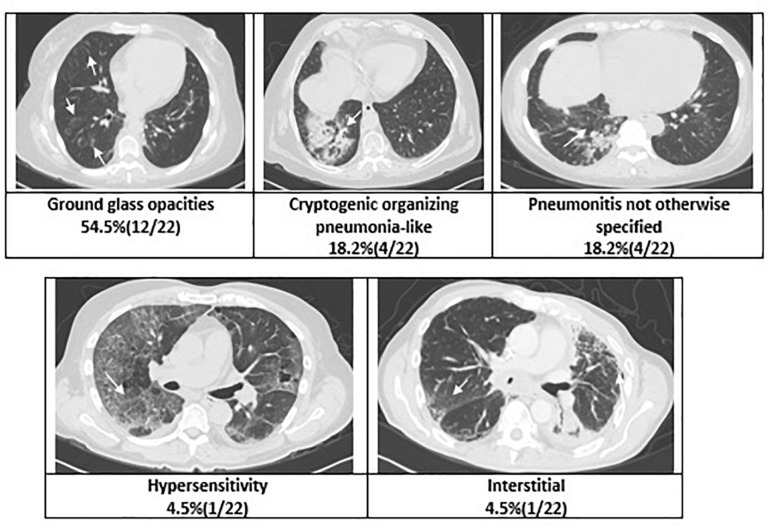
Radiographic patterns of pneumonitis.
